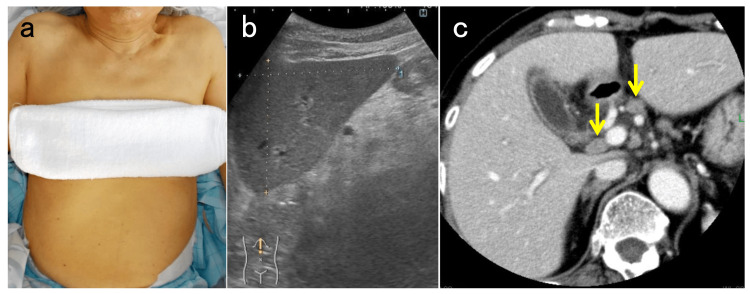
Skin appearance of the patient and findings of imaging studies of the abdomen. (a) Icteric skin is observed. (b) Ultrasound shows dullness of the edge of the left hepatic lobe, indicative of mild liver swelling. (c) On contrast-enhanced computed tomography, enlarged reactive lymph nodes (yellow arrows) are seen at the hepatic hilus.
